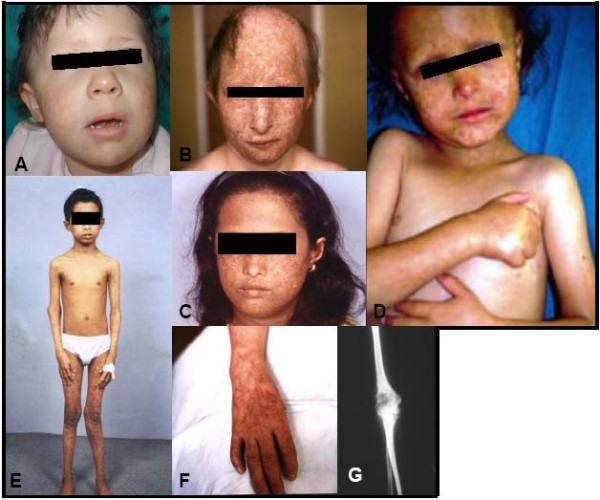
Panel showing some clinical features of the RTS syndrome. A) Chronic phase of cheek poikiloderma (4-year-old girl). B) Poikiloderma with alopecia (21-year-old boy). C) Poikiloderma. D) Poikiloderma sparing the trunk (courtesy of Professor M. Paradisi, Rome). E) Photo distributed poikiloderma and valgism of the knees. F) Thumb aplasia (patient B). G) Bone defect seen by X-Rays: *cystic*-like destructive lesion of the humerus (distal epiphysis) without apparent solution of continuity of the cortical bone (patient E).
